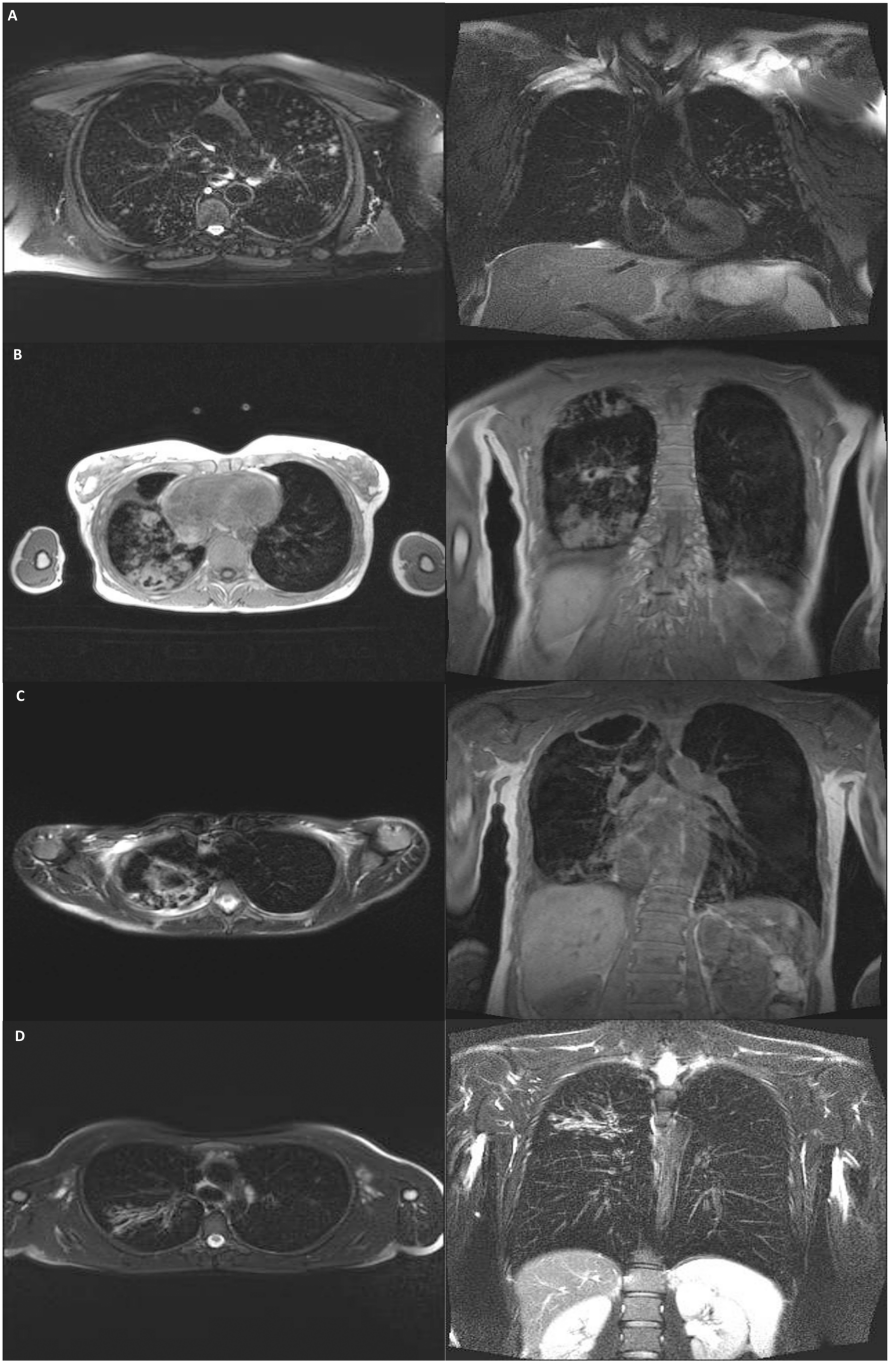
A: Thoracic MRI (T2 BLADE; transverse and coronal views) demonstrating bronchiectasis with branching nodular infiltrates in the left upper and right lower lobes, B: Thoracic MRI (VIBE; transverse and coronal views) demonstrating bronchiectasis with right upper and lower lobe cavities, nodular infiltrates, consolidation and pleural thickening, C: Thoracic MRI (VIBE; transverse and coronal views) demonstrating bronchiectasis with right upper lobe cavities with surrounding nodular infiltrates, D: Thoracic MRI (T2 BLADE; transverse and coronal views) demonstrating bronchiectasis with marked right upper lobe bronchial wall thickening and distal mucoid impaction.
